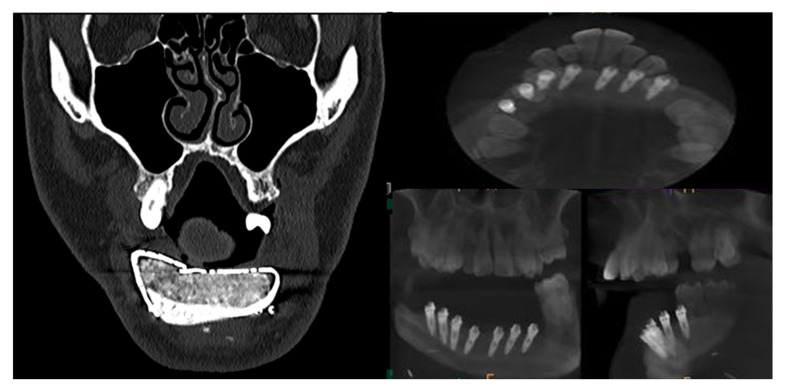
CT Scan and CBCT were performed in the postoperative follow-up, providing relevant quantitative data regarding bone volume and bone resorption.
